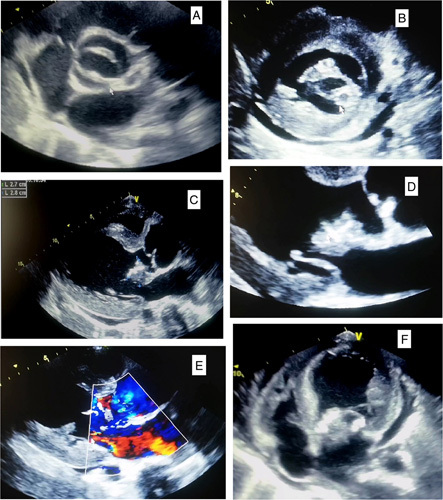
Short axis parasternal section showing bicuspid aortic valve (A),vegetation on the mitral valve (B). Long-axis parasternal section showing vegetation on the mitral and aortic valve (C, and D), mitro-aortic regurgitation (E) four-cavity section showing vegetation on the mitral valve (F).
